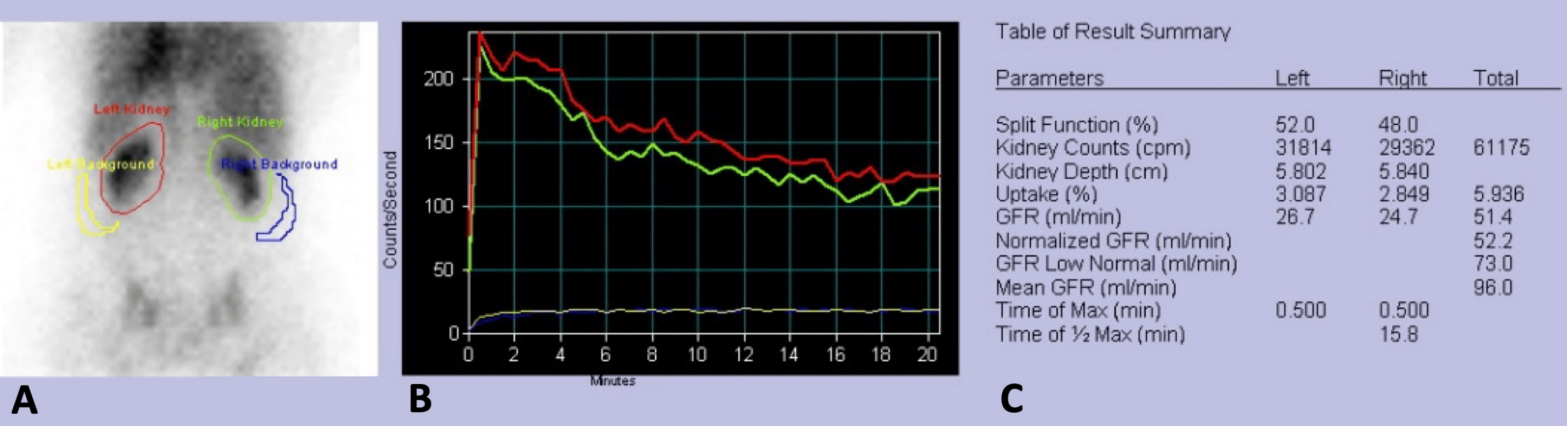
Renal scintigraphic study after intravenous administration of 99mTc-DTPA (technetium-99m-diethylene-triamine-pentaacetate) A static renal scintigraphy image (A), renogram (B), and result summary (C) GFR, glomerular filtration rate
